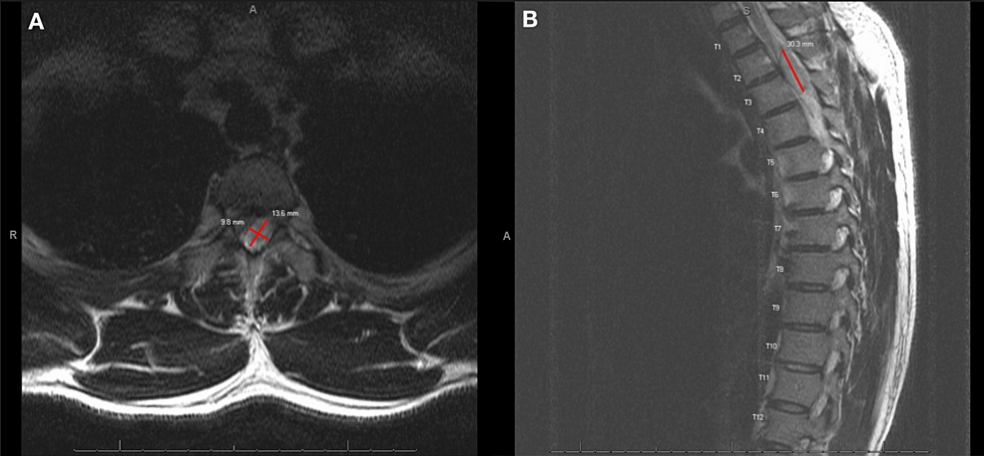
(A) Preoperative hypertense 3.0 cm extradural mass centered in the dorsal and left lateral canal at T2 on T2-weighted imaging on axial view highlighted in red. (B) Preoperative hyperintense 3.0 cm extradural mass centered in the dorsal and left lateral canal at T2 on T2-weighted imaging on sagittal view highlighted in red
